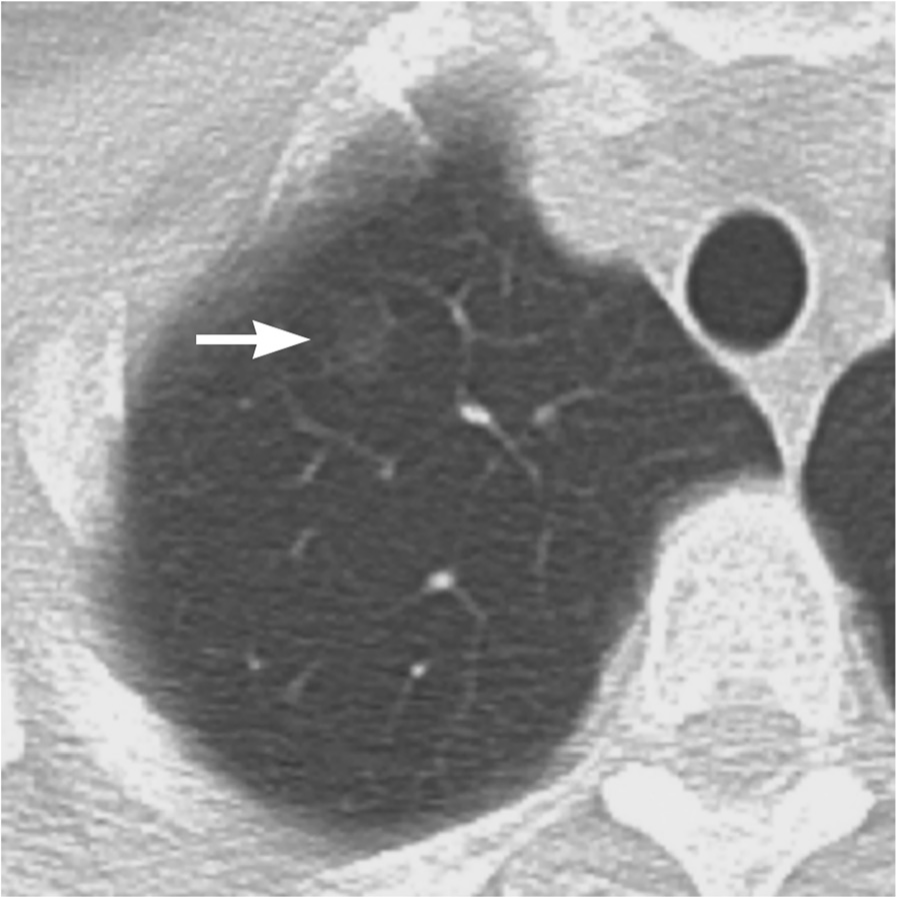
Screening chest CT at 2 years before CT examination at our hospital. A 7mm slice thickness CT shows a faint pure GGO in the right upper lobe (*arrow*), which is smaller than that of Figure 1.
